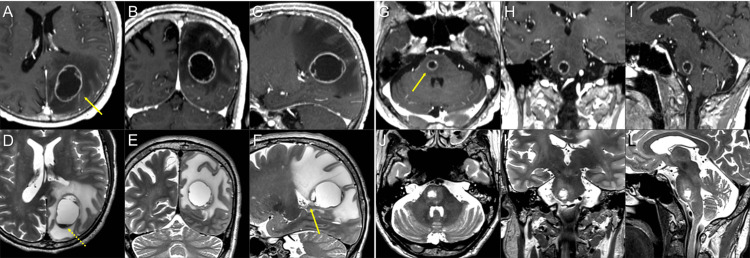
Magnetic resonance images for 10-fraction stereotactic radiosurgery planning. The images show contrast-enhanced (CE) T1-weighted images (T1-WI) (A-C, G-I); T2-weighted images (T2-WI) (D-F, J-L); axial images (A, D, G, J); coronal images (B, E, H, K); and sagittal images (C, F, I, L). (A-F) A cystic lesion (arrow in A) in the left parietal lobe is associated with massive surrounding edema and fluid sedimentation within the cyst (dashed arrow in D). The ventral side of the lesion is near the lateral ventricular wall (arrow in F). (G-I, J-L) A cystic lesion (arrow in G) in the pons with mild perilesional edema.
